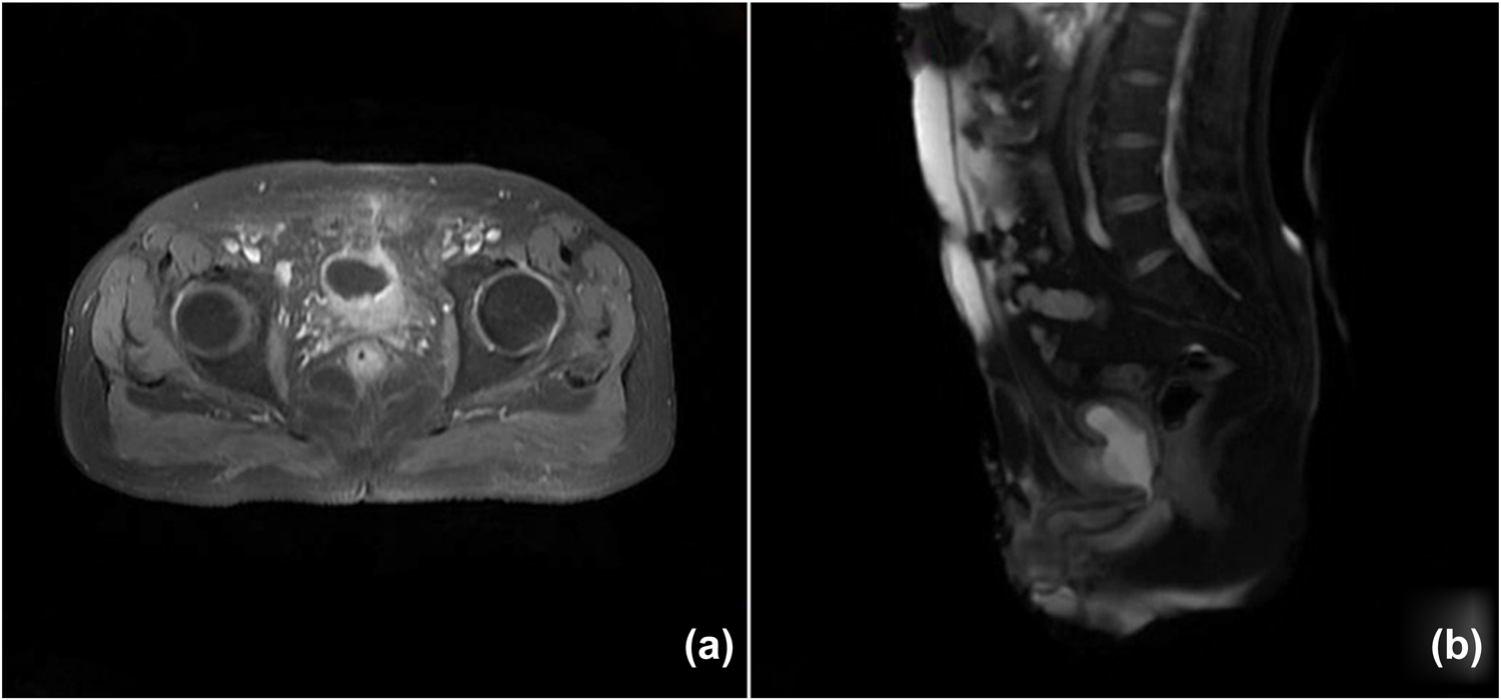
MRI of the pelvis confirming the presence of pubovesical fistula. Axial (a) and sagittal (b) images.
